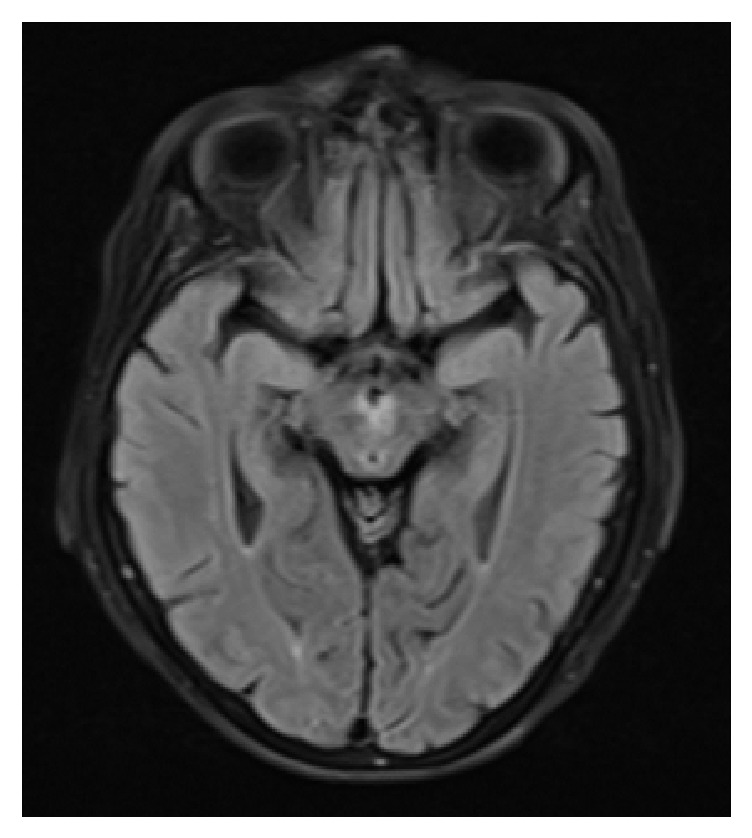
MRI brain with fluid attenuated inversion recovery (FLAIR) series demonstrating the “V sign” hyperintensity along the pial surface of the interpeduncular fossa in the midbrain.
